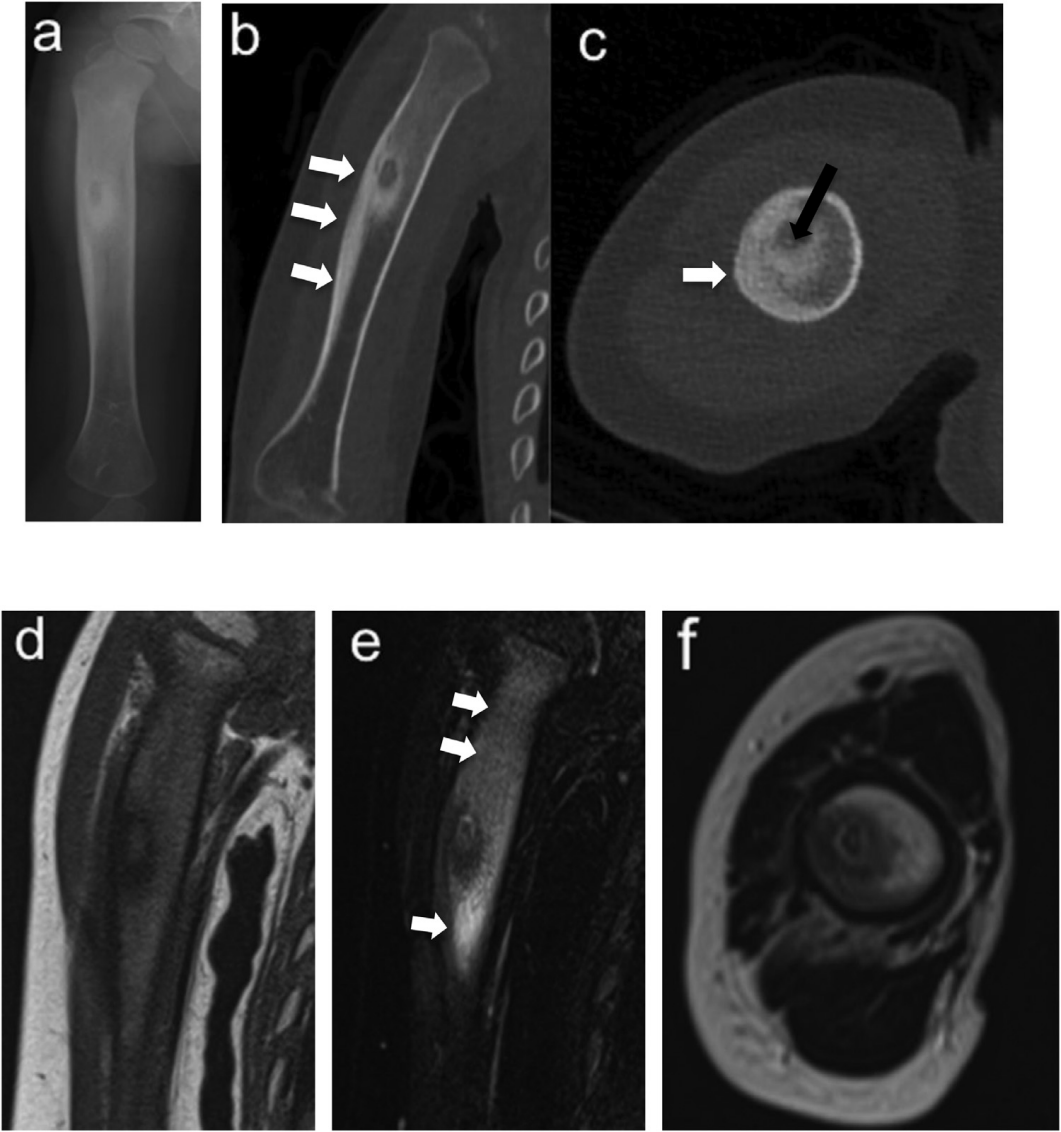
Radiograph, CT, and MRI of the right humerus at the patient's first visit (A: anteroposterior radiograph, B: coronal CT view, C: axial CT view, D: coronal view of T1-weighted MRI, E: coronal view of STIR MRI, F: axial view of T2-weighted MRI). The lucent area was located intramedullary, the nearby bone cortex was unilaterally thickened (white arrows in B, C), and a very fine calcification was observed inside the lucent area (black arrow in C). MRI on STIR showing exceedingly high intensity around the osteosclerotic zone which suggesting bone marrow edema (white arrows in E).
